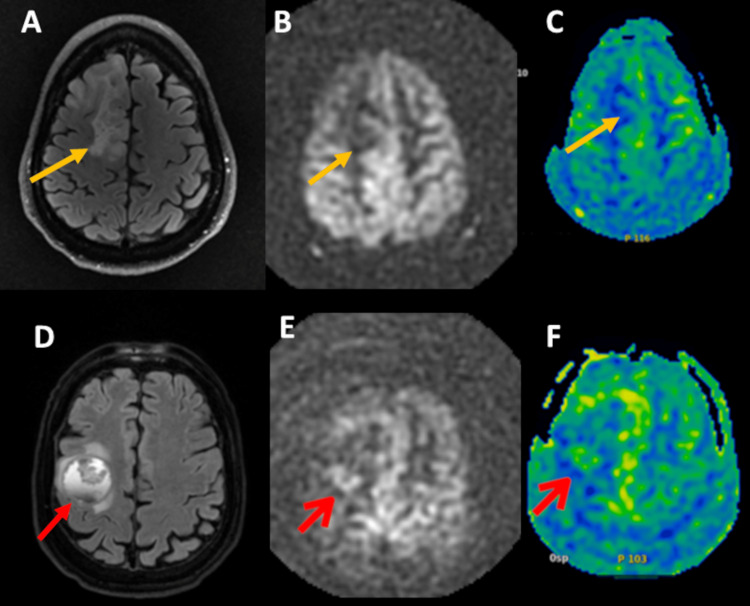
Role of ASL MRI in differentiating between different brain tumors. (A, B, C) The images represent a right frontal lobe oligodendroglioma (orange arrow). (D, E, F) The images represent right frontal lobe non-small cell lung cancer metastasis (red arrow). (A) FLAIR MRI shows a right frontal lobe oligodendroglioma. (B, C) axial pcASL (B) and color-coded CBF map (C) from pcASL data show no hyperperfusion. (D) FLAIR MRI shows a right frontal lobe non-small cell lung cancer metastasis with perilesional vasogenic edema. (E, F) axial pcASL (E) and color-coded CBF map (F) from pcASL data show heterogenous hyperperfusion amongst nodules at the super lesion margin.
